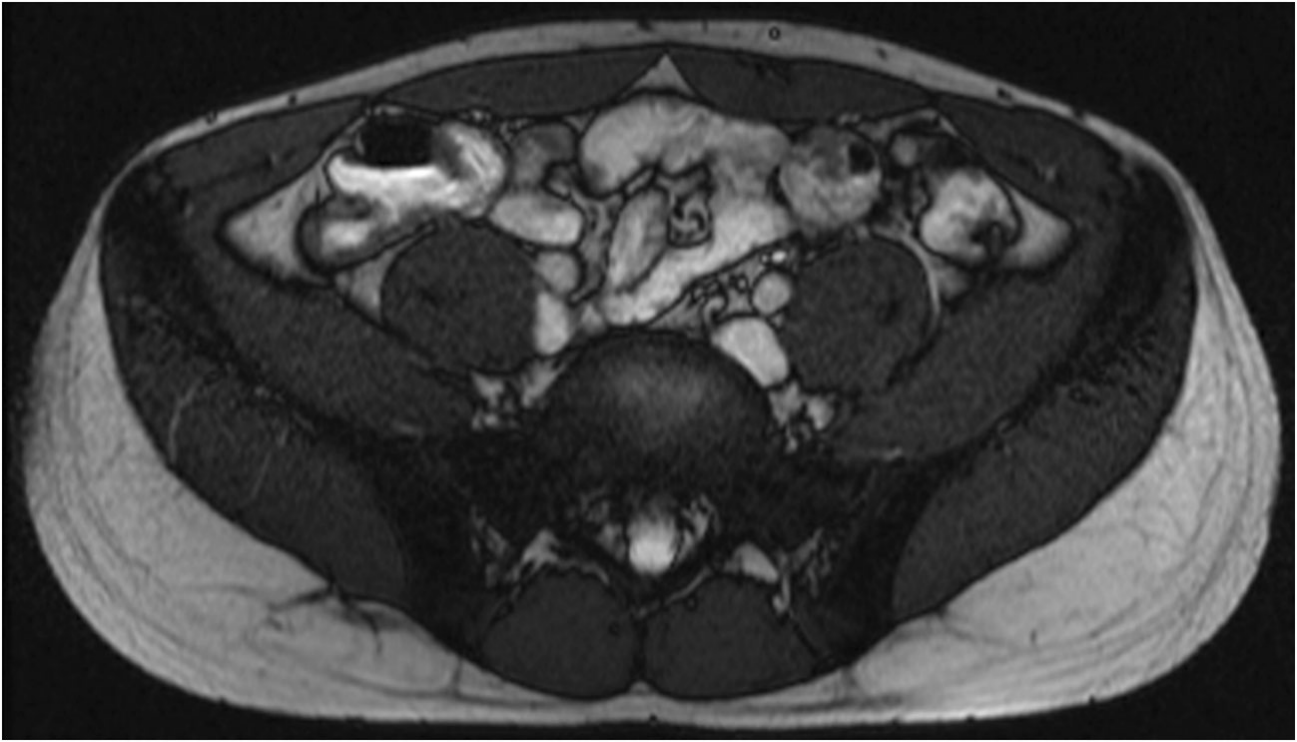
Markedly thickened (neo)terminal ileum with stenosis. Coronal T2-weighted post-contrast image of a patient with status after ileocaecal resection. MRI shows concentric wall thickening at the neoterminal ileum over a length of about 8 cm
